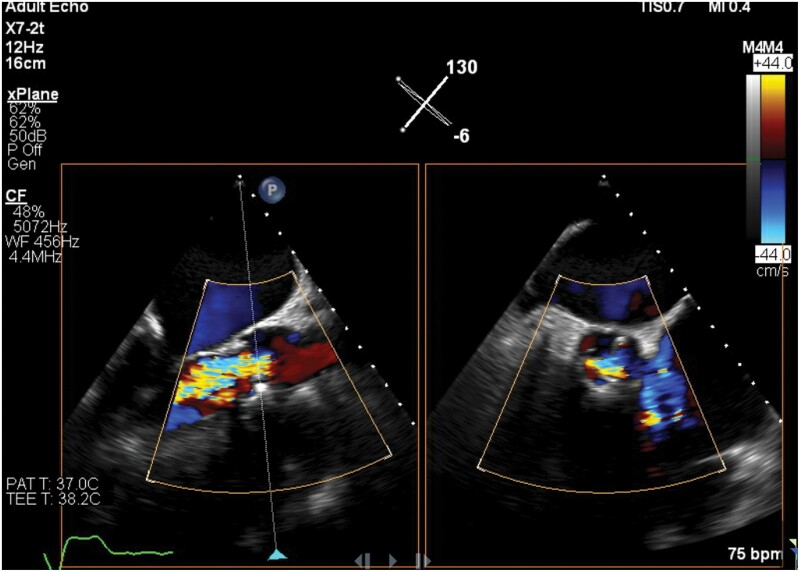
Echocardiographic images of central aortic regurgitation.
